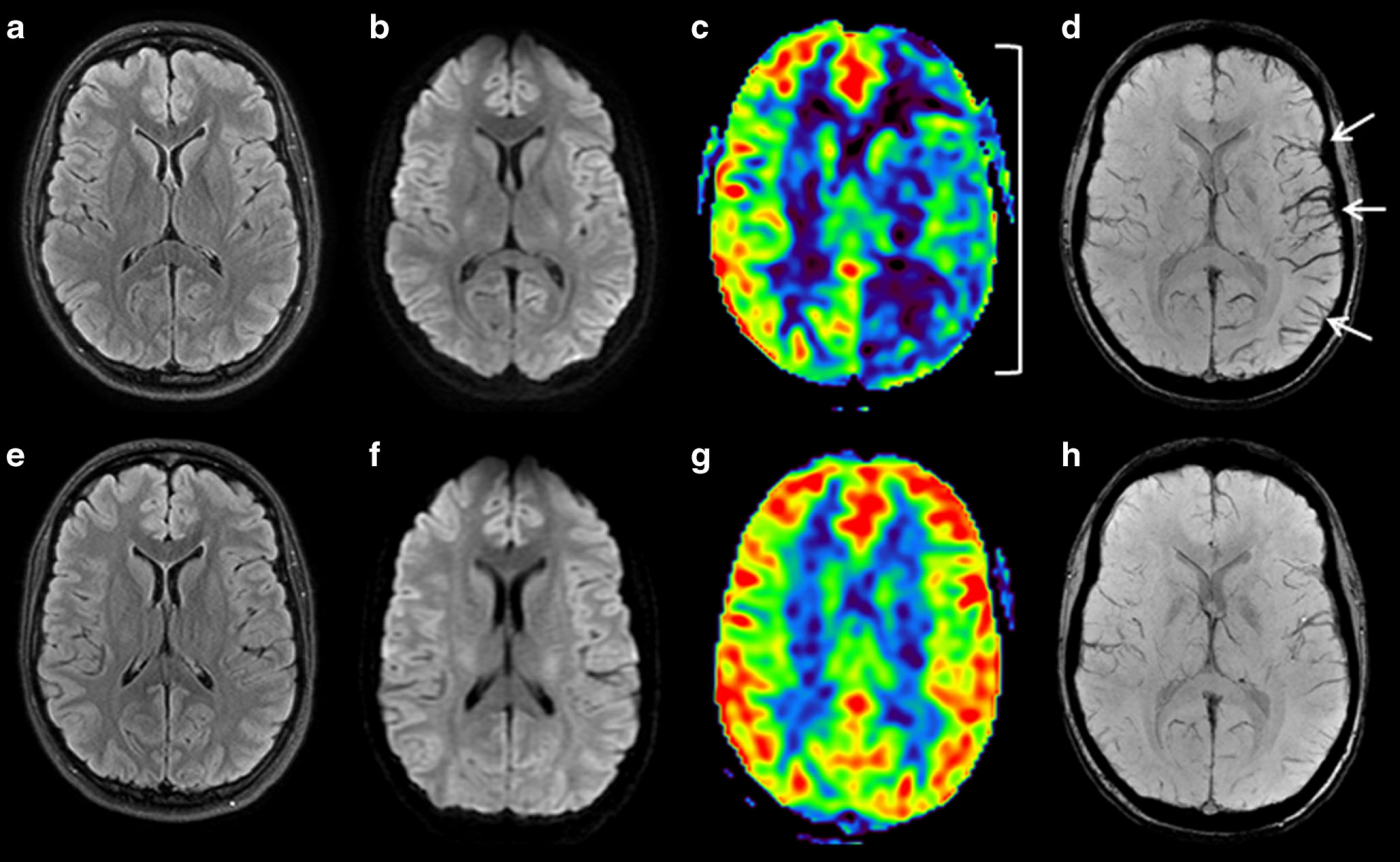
A 21-year-old patient presenting sudden-onset aphasia. Initial (*top row*) and control (*H24, bottom row*) brain MRI with FLAIR (a, e), DWI (b, f), ASL (c, g) and SWI (d, h) sequences in the axial plane. Initial MRI, a and b No visible acute ischaemic zone. c Large area of hypoperfusion affecting the whole left hemisphere (*parentheses*) related to migraine aura. d Left hemispheric brush sign (*white arrows*). Control MRI (H24) shows no ischaemic lesion (e and f), a normal left hemispheric perfusion (g), and a disappearance of the brush sign (h)
